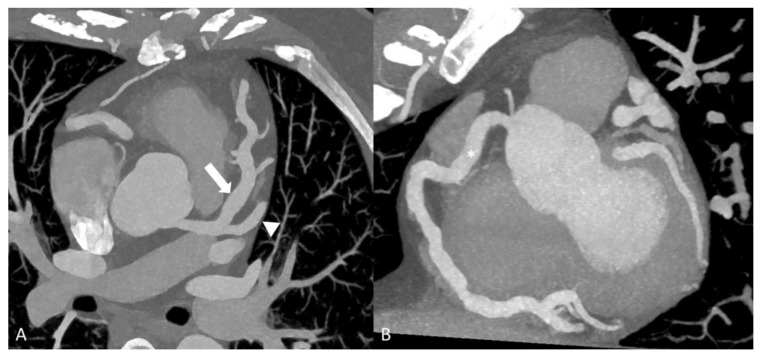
A 35-year-old woman with Kawasaki’s disease. Double oblique MPR images of coronary CTA (A,B) showing diffuse aneurysmal dilation to the LAD (arrow), large caliber first diagonal branch (arrowhead) and RCA (asterisk).
